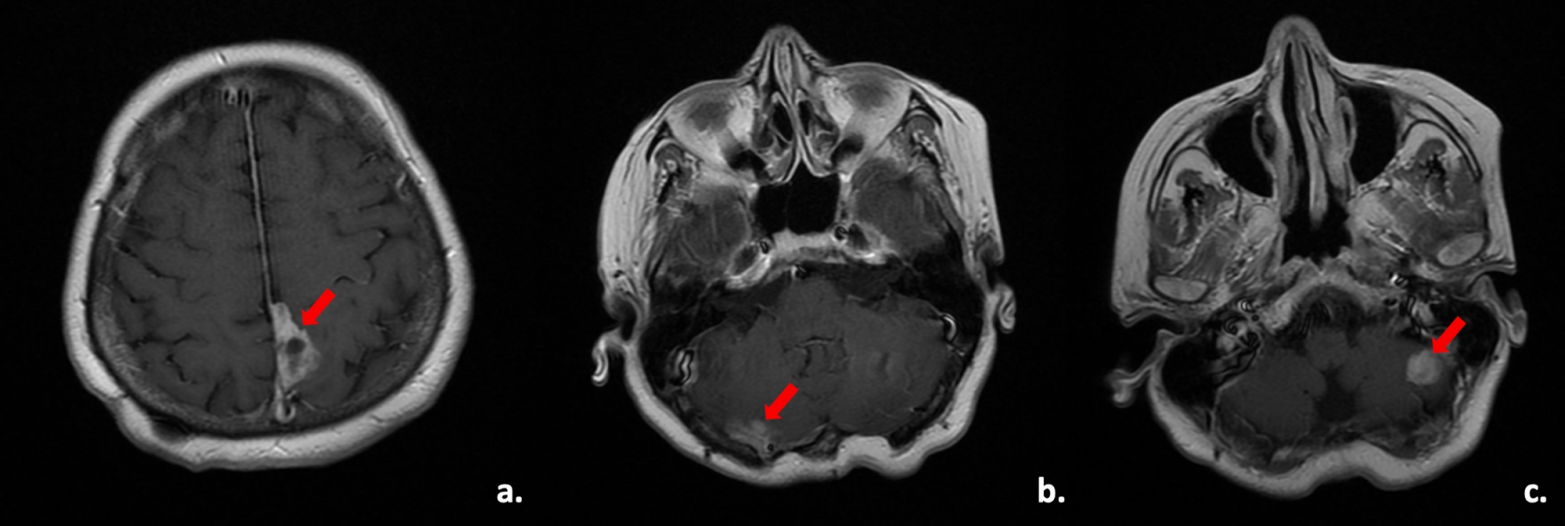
MRI scans of the lesions a. Axial contrast-enhanced magnetic resonance imaging (MRI) scan of the lesion in the left parietal lobe (right arrow). b and c. Axial contrast-enhanced MRI scan of the lesions in right and left cerebellar hemispheres (red arrows), respectively.
